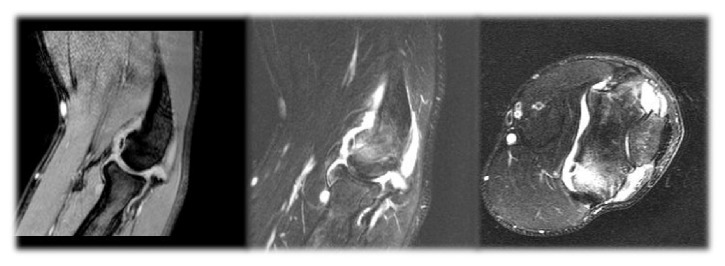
MRI of patients right elbow showing a small osteochondral capitellar lesion, intramedullary oedema, and an intra-articular loose body.
